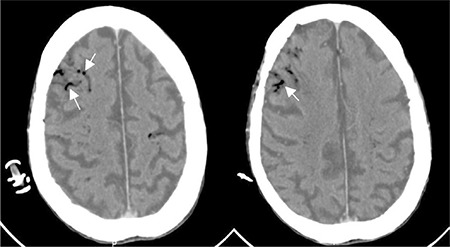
Unenhanced head computed tomography reveals cerebral air (white arrows) distributed in gyriform pattern in both cerebral hemispheres.
